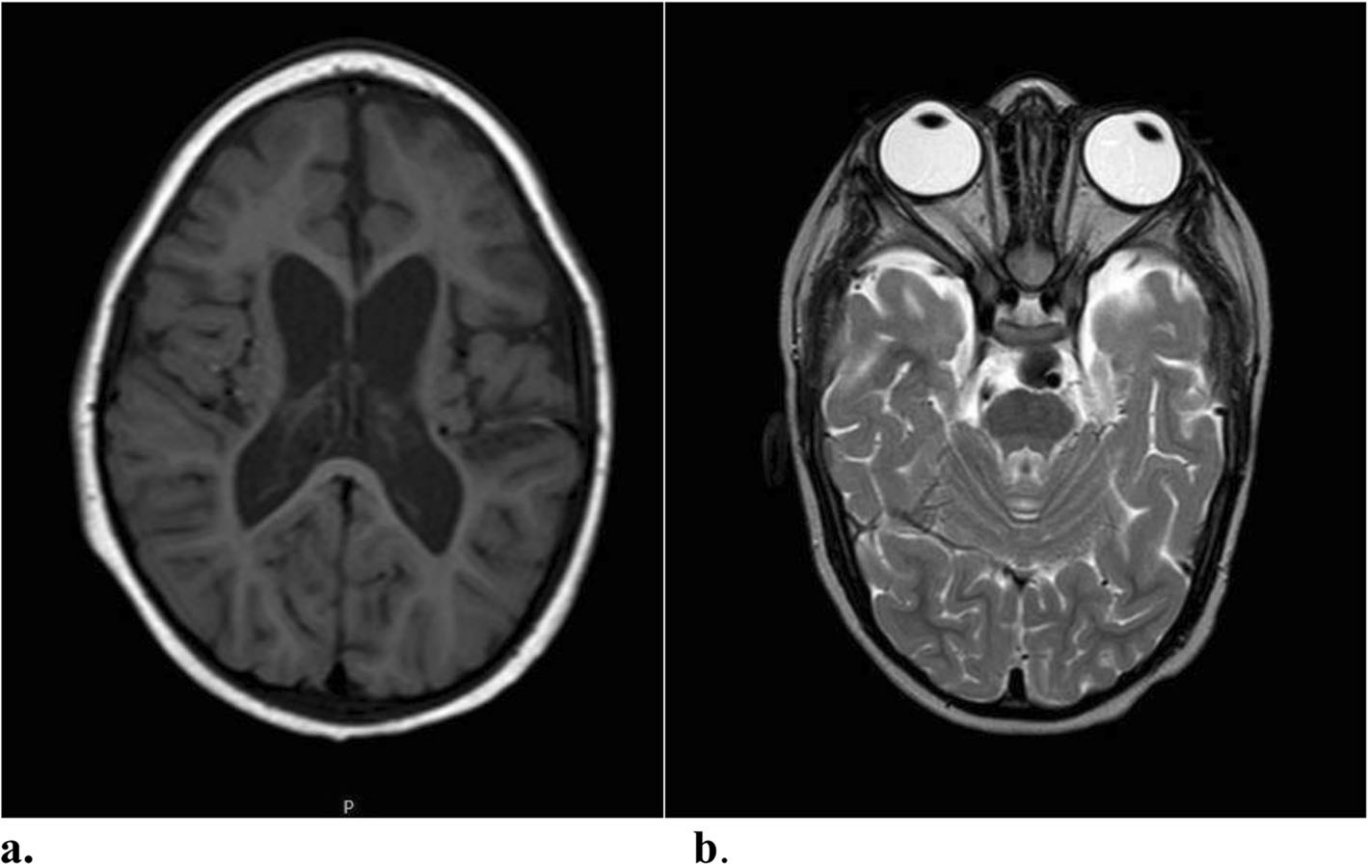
a-b: Brain MRI performed at 3 years: a) Axial T1-weighted image and b) Axial T2-weighted image: moderate enlargement of both lateral ventricles and subarachnoid space of bilateral temporal pole with volume reduction of the supratentorial white matter
